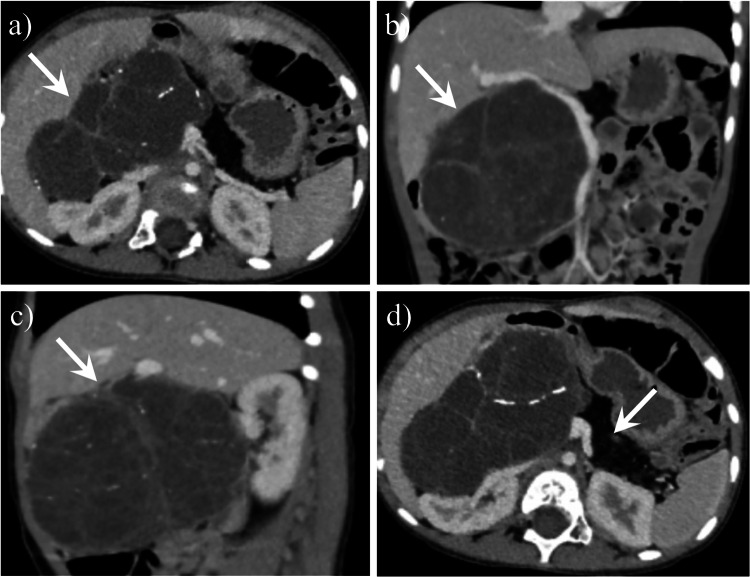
A 2-year-old girl with dorsal pancreatic agenesis coexisted with serous cystadenoma. Axial (a), coronal (b) and sagittal (c) contrast-enhanced computed tomography (CT) images revealed a large right intraperitoneal mass (arrow) with enhancing septage and central stellate calcification. Axial CT image (d) revealed the “absence” of the pancreatic body-tail portion, and the corresponding region showed fat density (arrow). This is the second case of a serous cystadenoma of the pancreas occurring in the context of dorsal pancreatic agenesis
